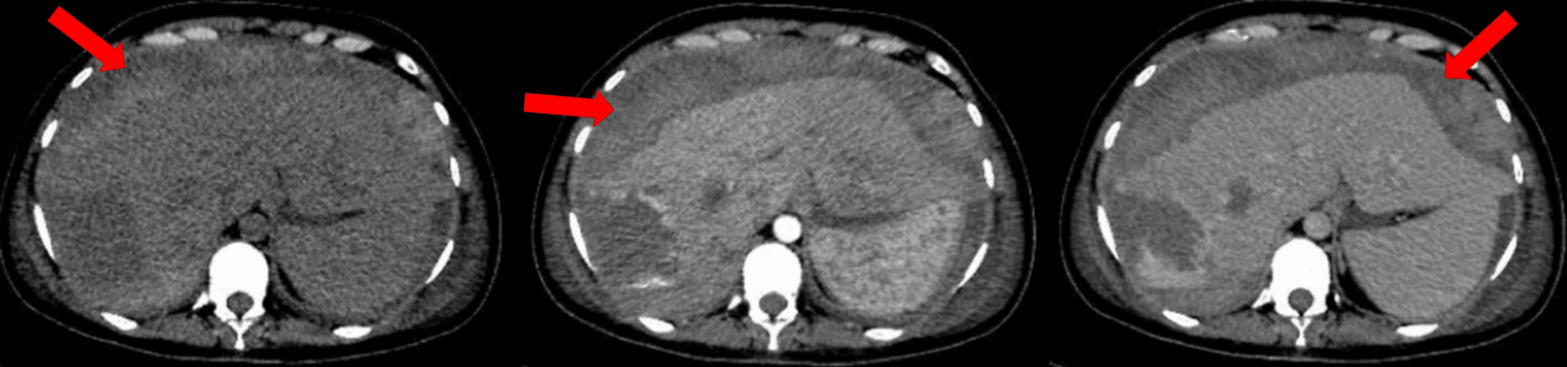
Postoperative computed tomography shows a large hepatic subcapsular hematoma
